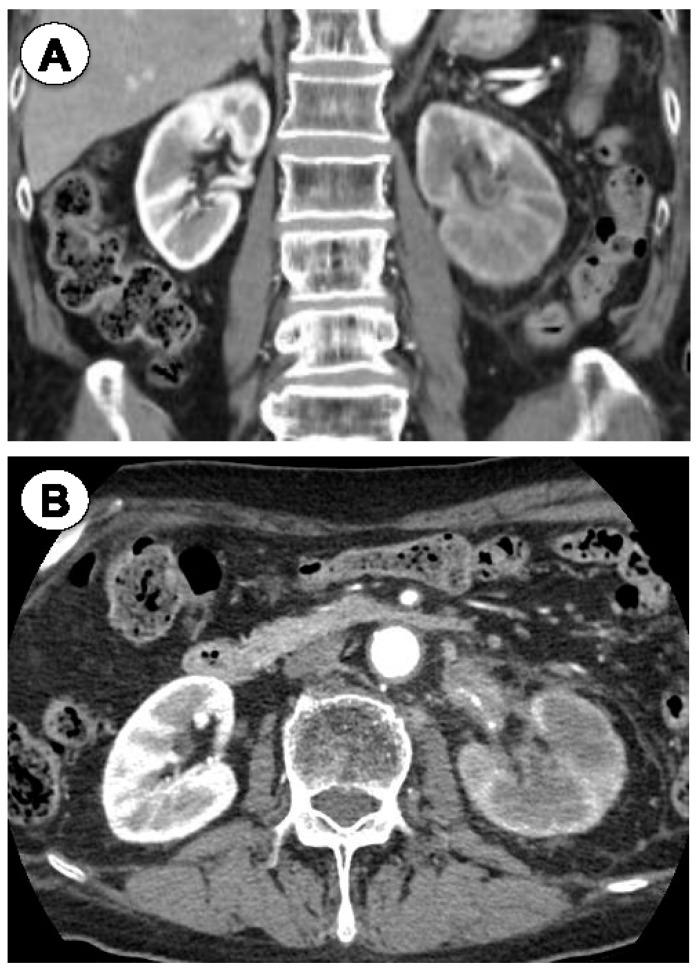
Case 2. Coronal (A) and axial (B) CT images demonstrating invasive left renal pelvic cancer.
